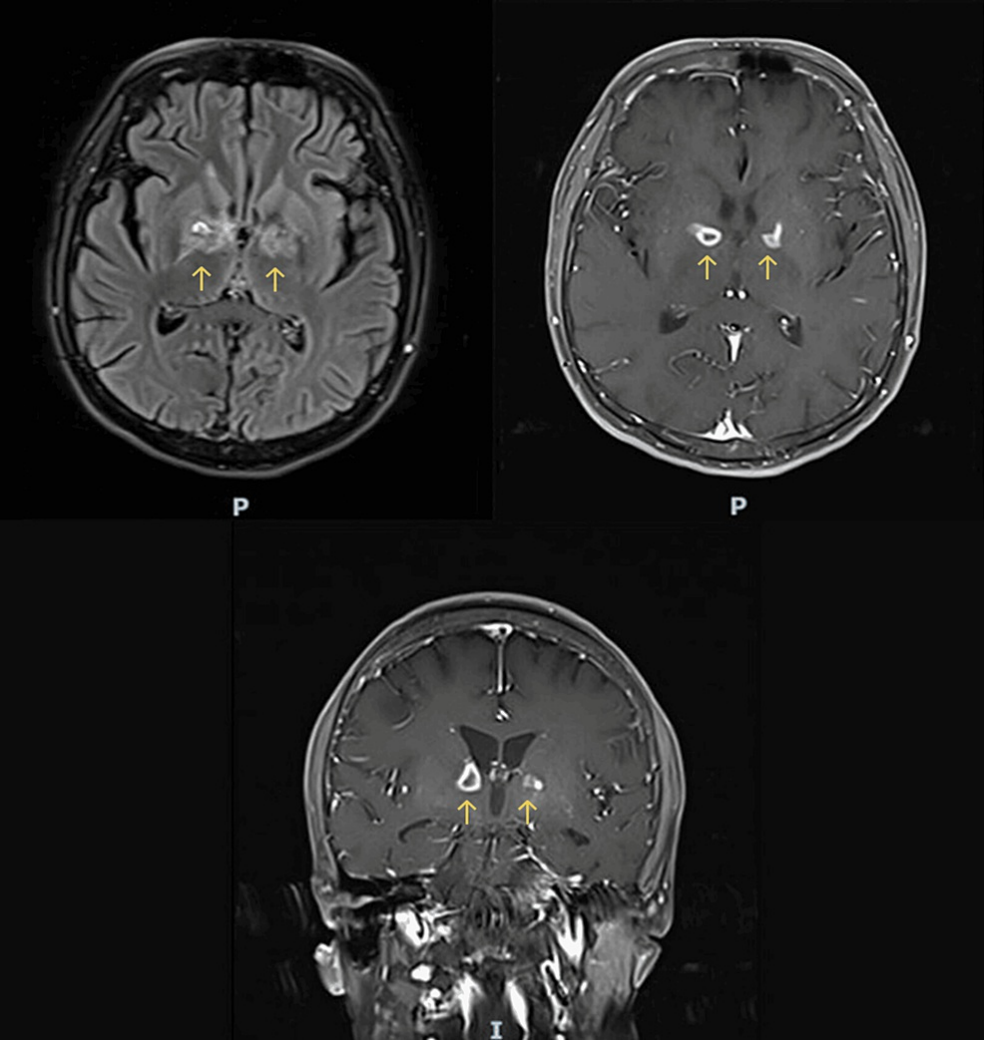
Repeat brain MRI showing significant improvement with marked regression of bilateral basal ganglia ring-enhancing lesions as well as reduction of edema MRI: magnetic resonance imaging
